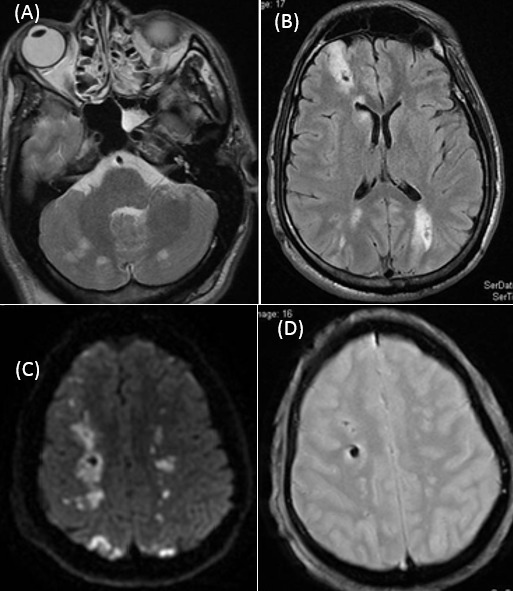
(A, B, C) white matter changes. The first examination, 3 days after the onset of cerebral malaria, shows diffuses hyperintensities on T2, T2- FLAIR-weighted and the SWI sequences images in the centrum semiovale, periventricular, frontal and occipital white matter, and in the cerebellum; (D) with central microhemorrhages in the occipital, frontal and right semiovale centrum white matter lesions on Gradient Echo (T2*) sequence
